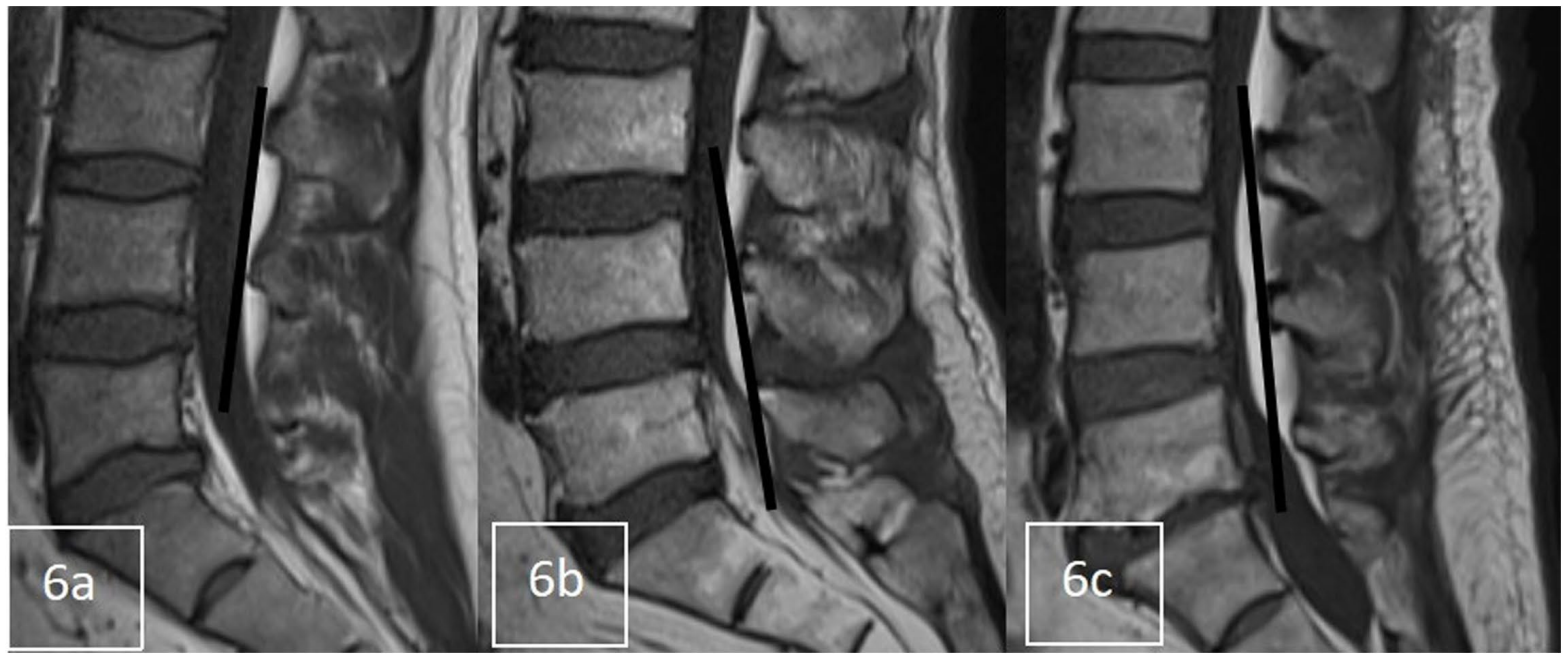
Sagittal grading of epidural fat on T1-weighted MR images. (A) Grade 1 was defined as epidural fat observed within the border between the anterosuperior edges of the upper and lower neighboring neural arches. (B) Grade 2 was defined as fat observed over the border at the middle but not at the edges of neural arches on both sides. (C) Grade 3 was defined as fat observed over the border at the edges of neural arches on at least one side.
